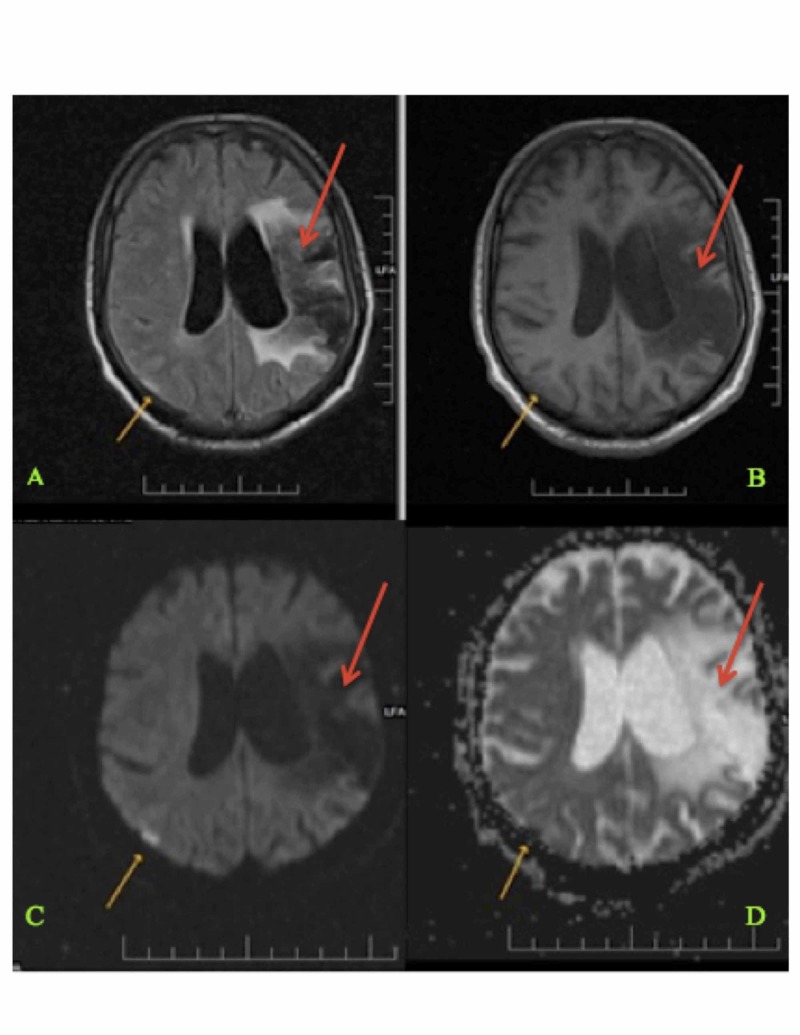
Magnetic Resonance Imaging (MRI) of the Brain Showing Current and Previous Infarction Sites (Panels A-D) Magnetic resonance imaging (MRI) of the brain showing an acute right-sided parietal lobe infarct (shown with yellow arrows) and an old left-sided middle cerebral artery territory infarct (shown with red arrows) in a 63-year-old patient [9].
